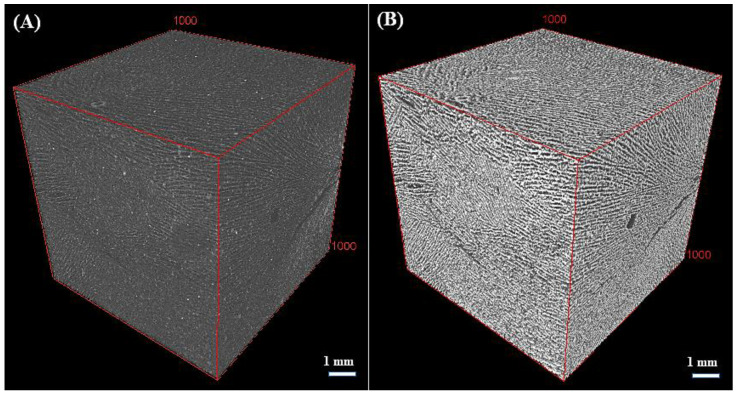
Example of FG20 sample segmentation using LABKIT plug-in. (A) Original X-ray CT image, and (B) binary image.
